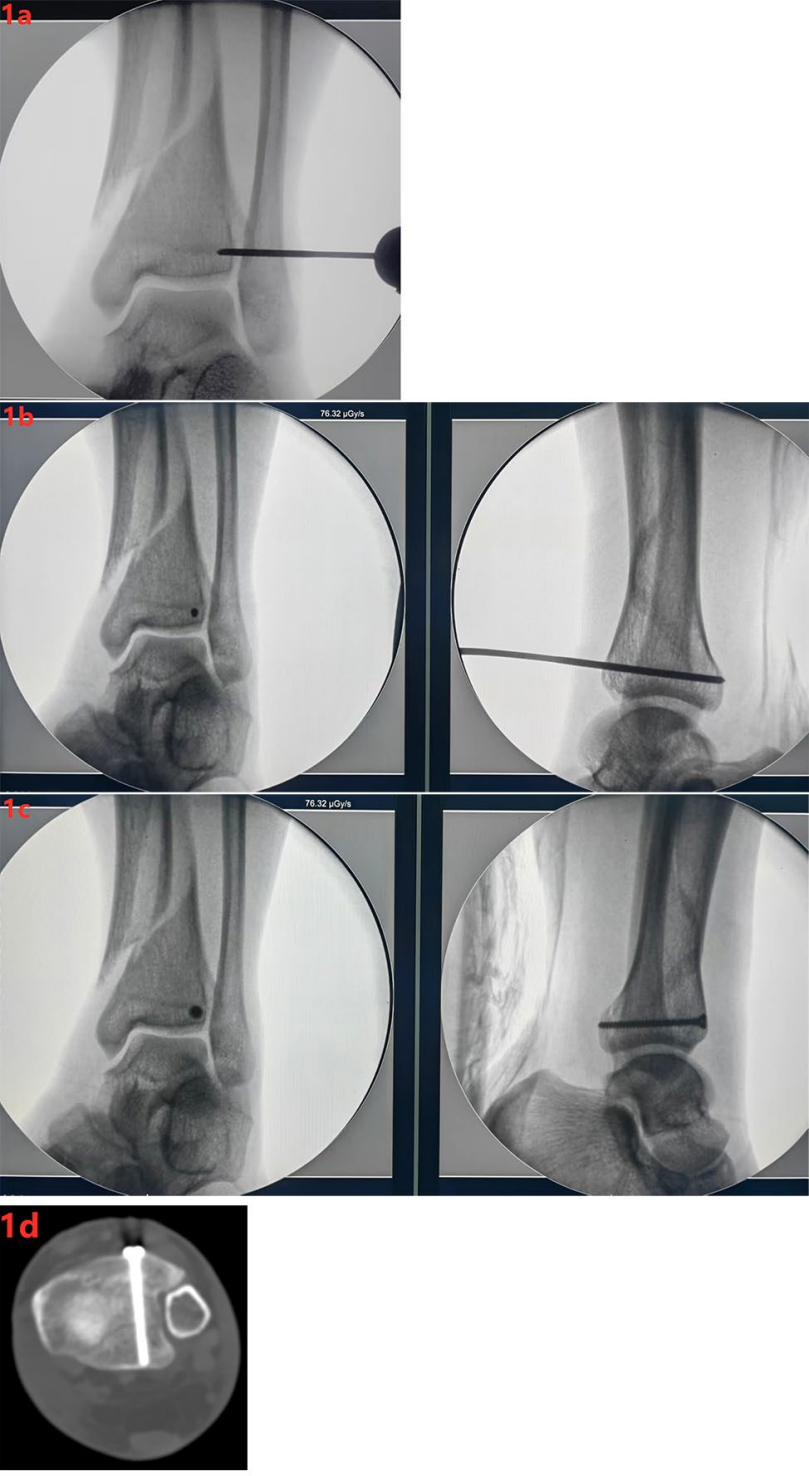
(a) X-ray films of standard ankle acupoints were projected by c-arm machine to locate the insertion point of screw channel; (b) Use a 2.5 mm Kirschner pin as the drill bit to drill the screw channel. After the position of the channel is confirmed by fluoroscopy, measure the length of the screw channel and tap; (c) Screw the screw into the channel for fixation (the channel direction is roughly parallel to the direction of the lower tibiofibular notch in the anteroposterior position, while in the lateral X-ray film, it should be consistent with the posterior inclination of the ankle joint); (d) Image of screw on CT cross section
